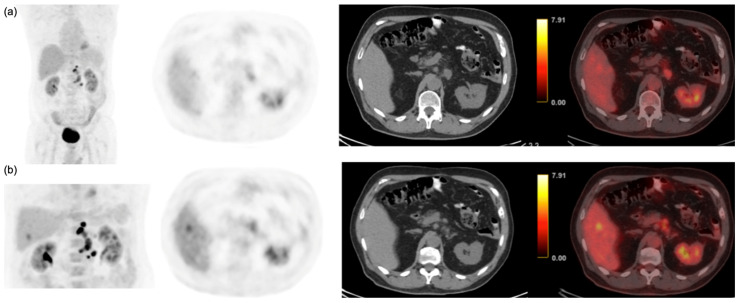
68-year-old patient with colorectal cancer and no liver lesion detected on contrast-enhanced computed tomography. (a) Coronal MIP, axial PET, axial CT, and fused CT during the early phase did not demonstrate any hypermetabolic focal liver lesion; (b) Coronal MIP, axial PET, axial CT, and fused images during the delayed phase demonstrate a focal hypermetabolic lesion in hepatic segment 5 corresponding to a liver metastasis.
